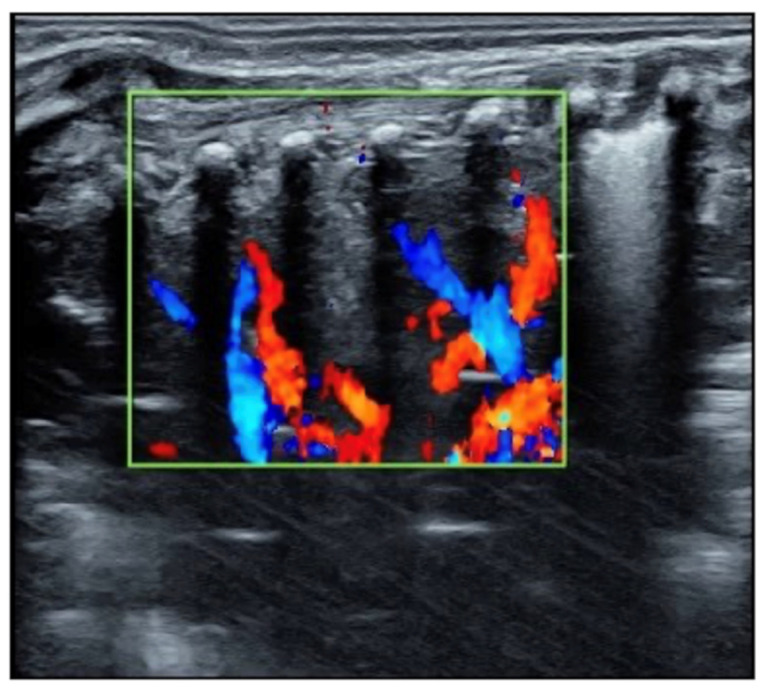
Blood supply in the consolidated lung area; Doppler ultrasound showed that the blood supply existed within the consolidated area. The presence of blood supply in the lung tissue of consolidation is a prerequisite for the recovery of severe atelectasis.
